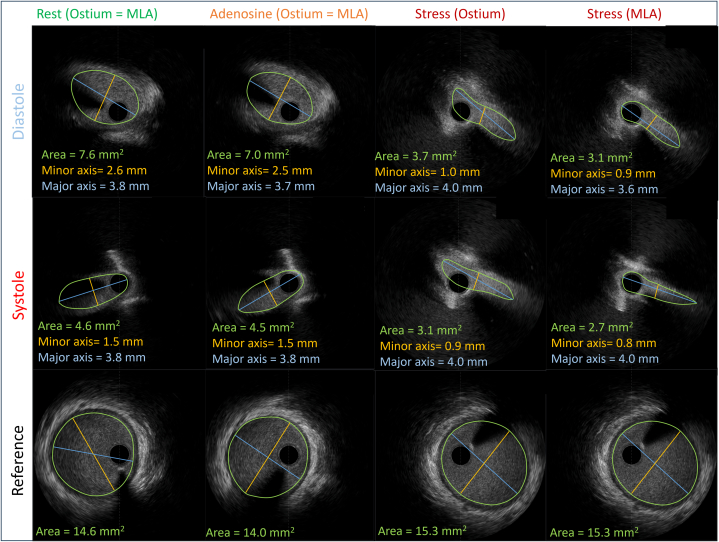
IVUS Ostial Cross-Sections (Diastole and Systole) at Rest, During Adenosine, and During Dobutamine Stress (First Column) At rest: diastolic lumen area 7.6 mm^2^ and systolic lumen area 4.6 mm^2^ (reference area: 14.6 mm^2^). (Second Column) Adenosine: diastolic 7.0 mm^2^ and systolic 4.5 mm^2^ (reference area: 14.0 mm^2^). (Third Column) Dobutamine volume challenge (stress ostium): diastolic 3.7 mm^2^ and systolic 3.1 mm^2^. (Fourth Column) Dobutamine volume challenge (stress ostium): diastolic 3.1 mm^2^ and systolic 2.7 mm^2^. Note the marked reduction in diastolic lumen area during stress compared with at rest (7.6 → 3.7 mm^2^). Panels are aligned to the same scale. Lumen contours are in green, minor axis is in yellow, and the major axis is in blue. IVUS = intravascular ultrasound; MLA = minimal lumen area.
